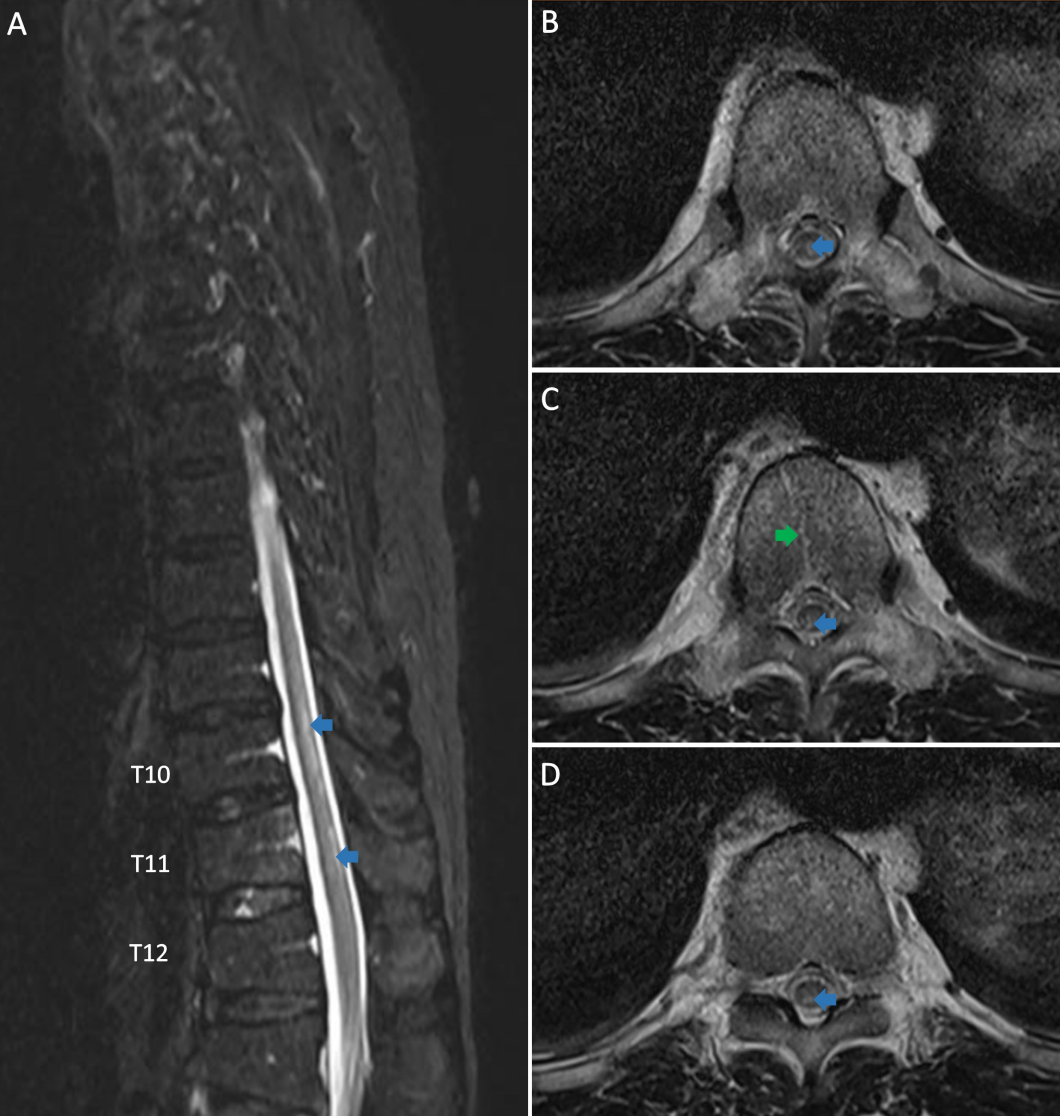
The T2-weighted magnetic resonance imaging of thoracic spine is shown, three days after symptoms onset. A: the sagittal image and B, C, D: the axial images showing hyperintensity in the posterior and posterior-central aspects of the thoracic spinal cord (blue arrows) and T2-weighted hyperintensity with enhancement is seen affecting T11 vertebra (green arrows).
